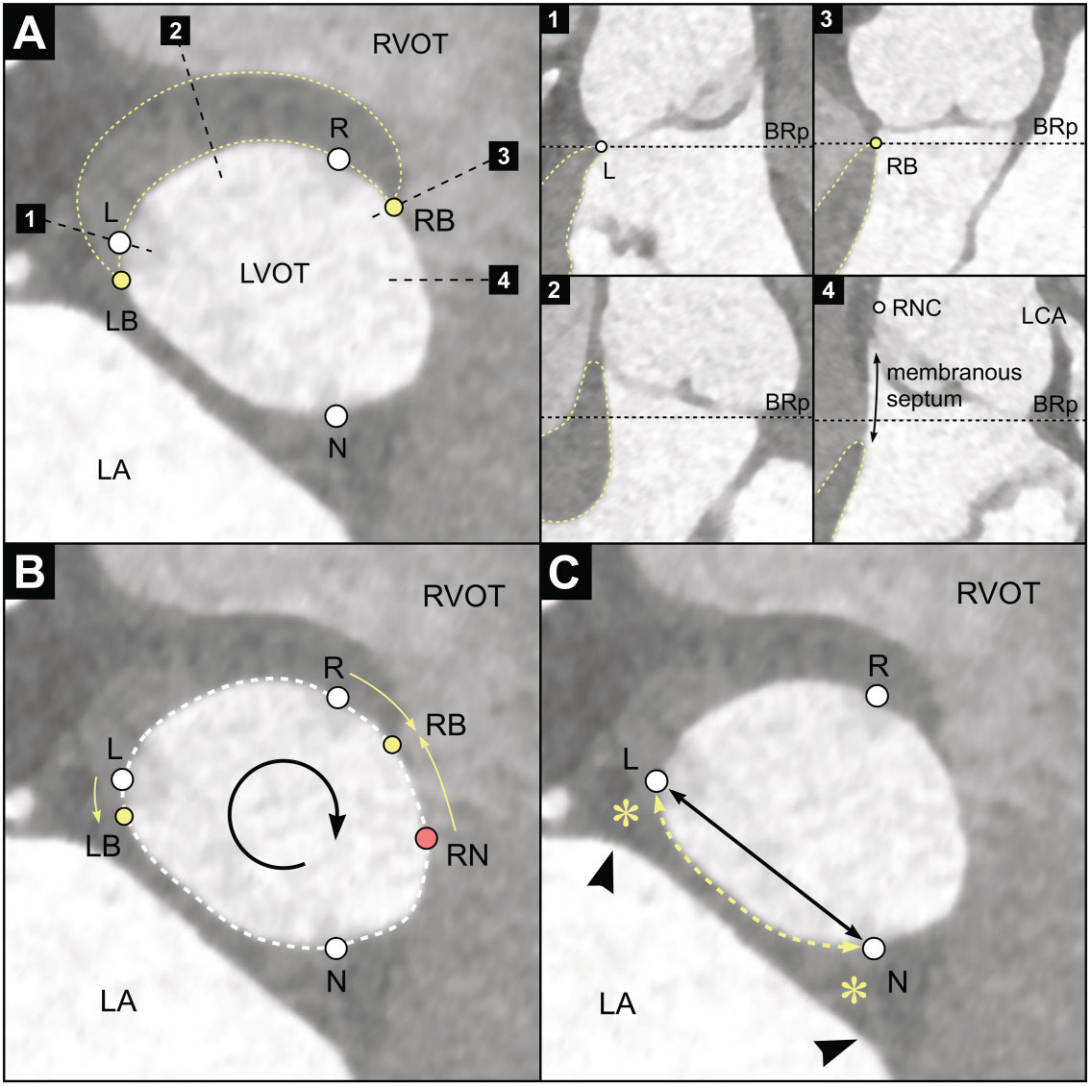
Computed tomography angiography of the aortic root at the level of the basal ring. (A) White points labelled L, R and N mark the nadirs of the left, right and non-coronary sinuses, respectively. The yellow dotted line encircles the left ventricular muscle. Yellow points labelled LB and RB mark the left and right border of the muscular part of basal ring, respectively. Black dotted lines with numbers show locations of perpendicular vertical transections through the basal ring plane, which are shown on the right as sub-Figs 1–4. In the sub-figures, the black dotted line shows the location of the basal ring plane (BRp). (B) Measured distances along the basal ring perimeter. We measured distances between the left (LB) and right (RB) border of the muscular part of BR and the nadirs of the left (L) and right (R) sinuses and the projection of the right-non commissure to the basal ring (RN). Distances are positive in the clockwise direction viewed from the aortic root and negative in the counterclockwise direction. In this patient, the L–LB distance is –1.7 mm, R–RB distance 6.5 mm and RNC-RB distance –8.7 mm. (C) Estimates of intertrigonal distance. Intertrigonal distance was estimated by direct distance between left (L) and non-coronary (N) nadir (black line with arrows) and distance along BR perimeter between left and non-coronary nadir (yellow dashed line with arrows). Asterisks mark the left and right fibrous trigones. Arrowheads mark external landmarks for the trigones seen as indentations when viewed from the LA. LA: left atrium; LCA: left coronary artery; LVOT: left ventricular outflow tract; RNC: right-non commissure; RVOT: right ventricular outflow tract.
